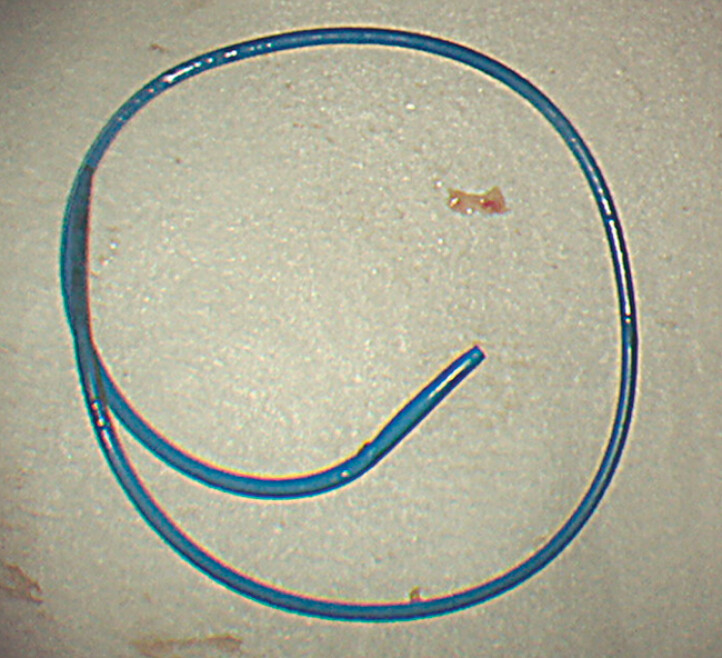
Successfully retrieved migrated plastic stent from a 55-year-old Japanese man with a pelvic abscess secondary to a hepatic abscess who underwent endoscopic ultrasound-guided pelvic abscess drainage.
